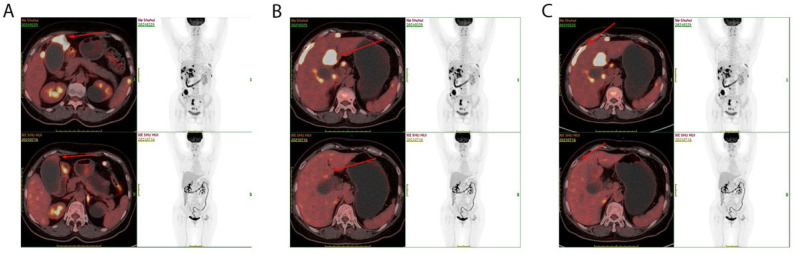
Positron emission tomography/computed tomography (PET/CT). (A) Glucose metabolism of the mass at the bottom of the gallbladder was decreased. (B) Glucose metabolism in the liver parenchyma around the gallbladder wall was decreased. (C) Glucose metabolism of multiple abdominal nodules surrounding liver capsule was decreased. The upper images (A–C) are the baseline images of PET/CT in February 2021, and the lower images (A–C) are the follow-up images of PET/CT in July 2021.
